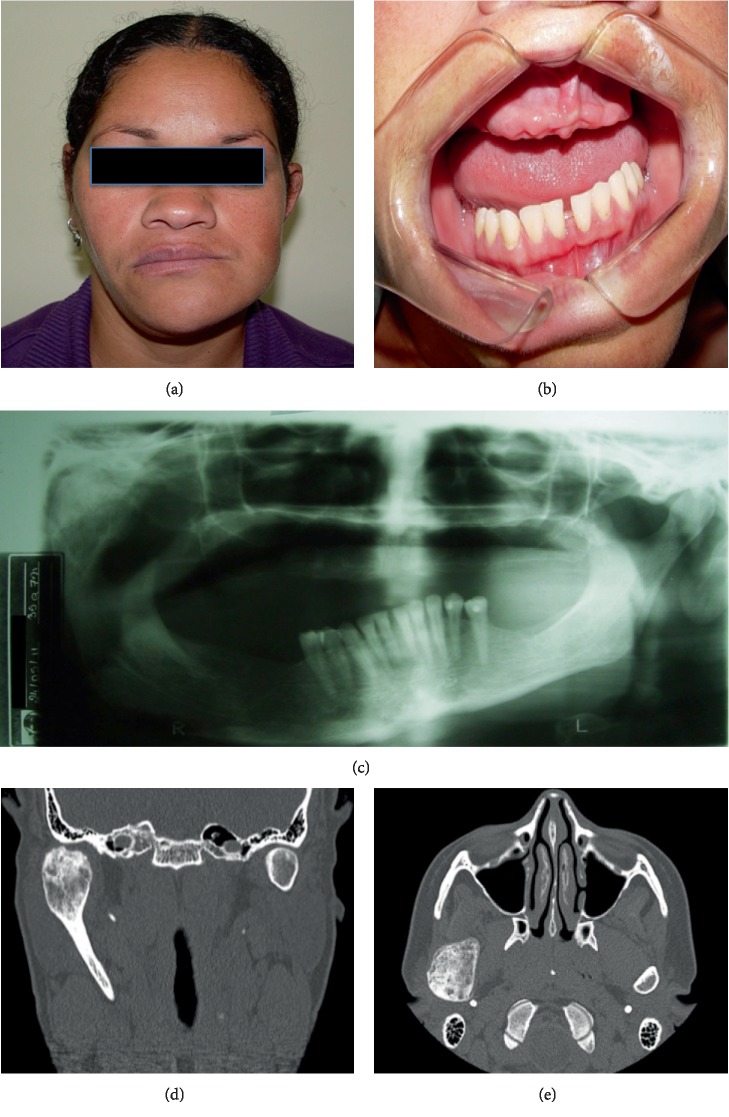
(a) Clinical facial aspect before surgery. The patient presented facial elongation, with a left chin deviation. (b) Occlusal plane alteration of the mandible. Patient with an edentulous maxilla. (c) Panoramic RX showing a tumoral mass associated to the right mandibular condyle. (d, e) Coronal and axial CT planes showing the size and limits of the tumor.
